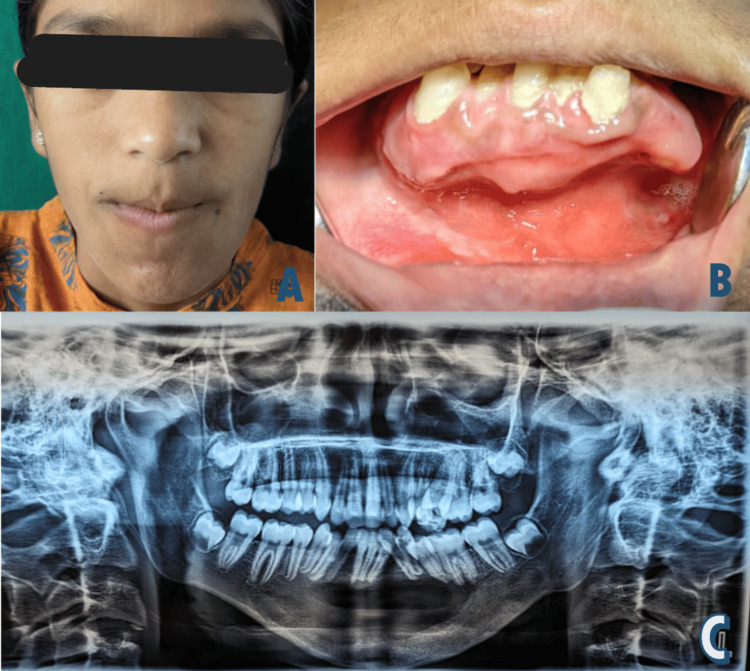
Postoperative clinical and radiographical pictures of the case A) Clinical picture of the one-year follow-up. B) Intraoral picture showing healing granulation tissue on one-year follow-up. C) Orthopantamogram at the one-year follow-up, with good healing and no signs of recurrence or infection. Written informed consent to publish the patient's image in an open-source journal has been obtained from the patient's legal guardian.
